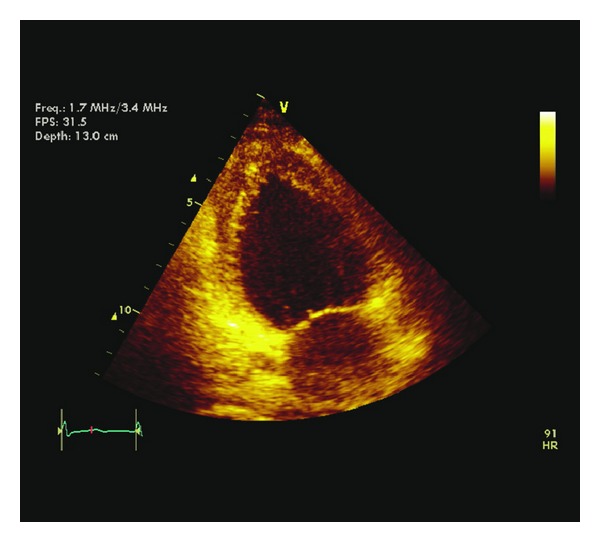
Apical 2-chamber view during systole showing basal hypokinesis and normal apex, the “ace of spades” appearance of left ventricular contractile function.
